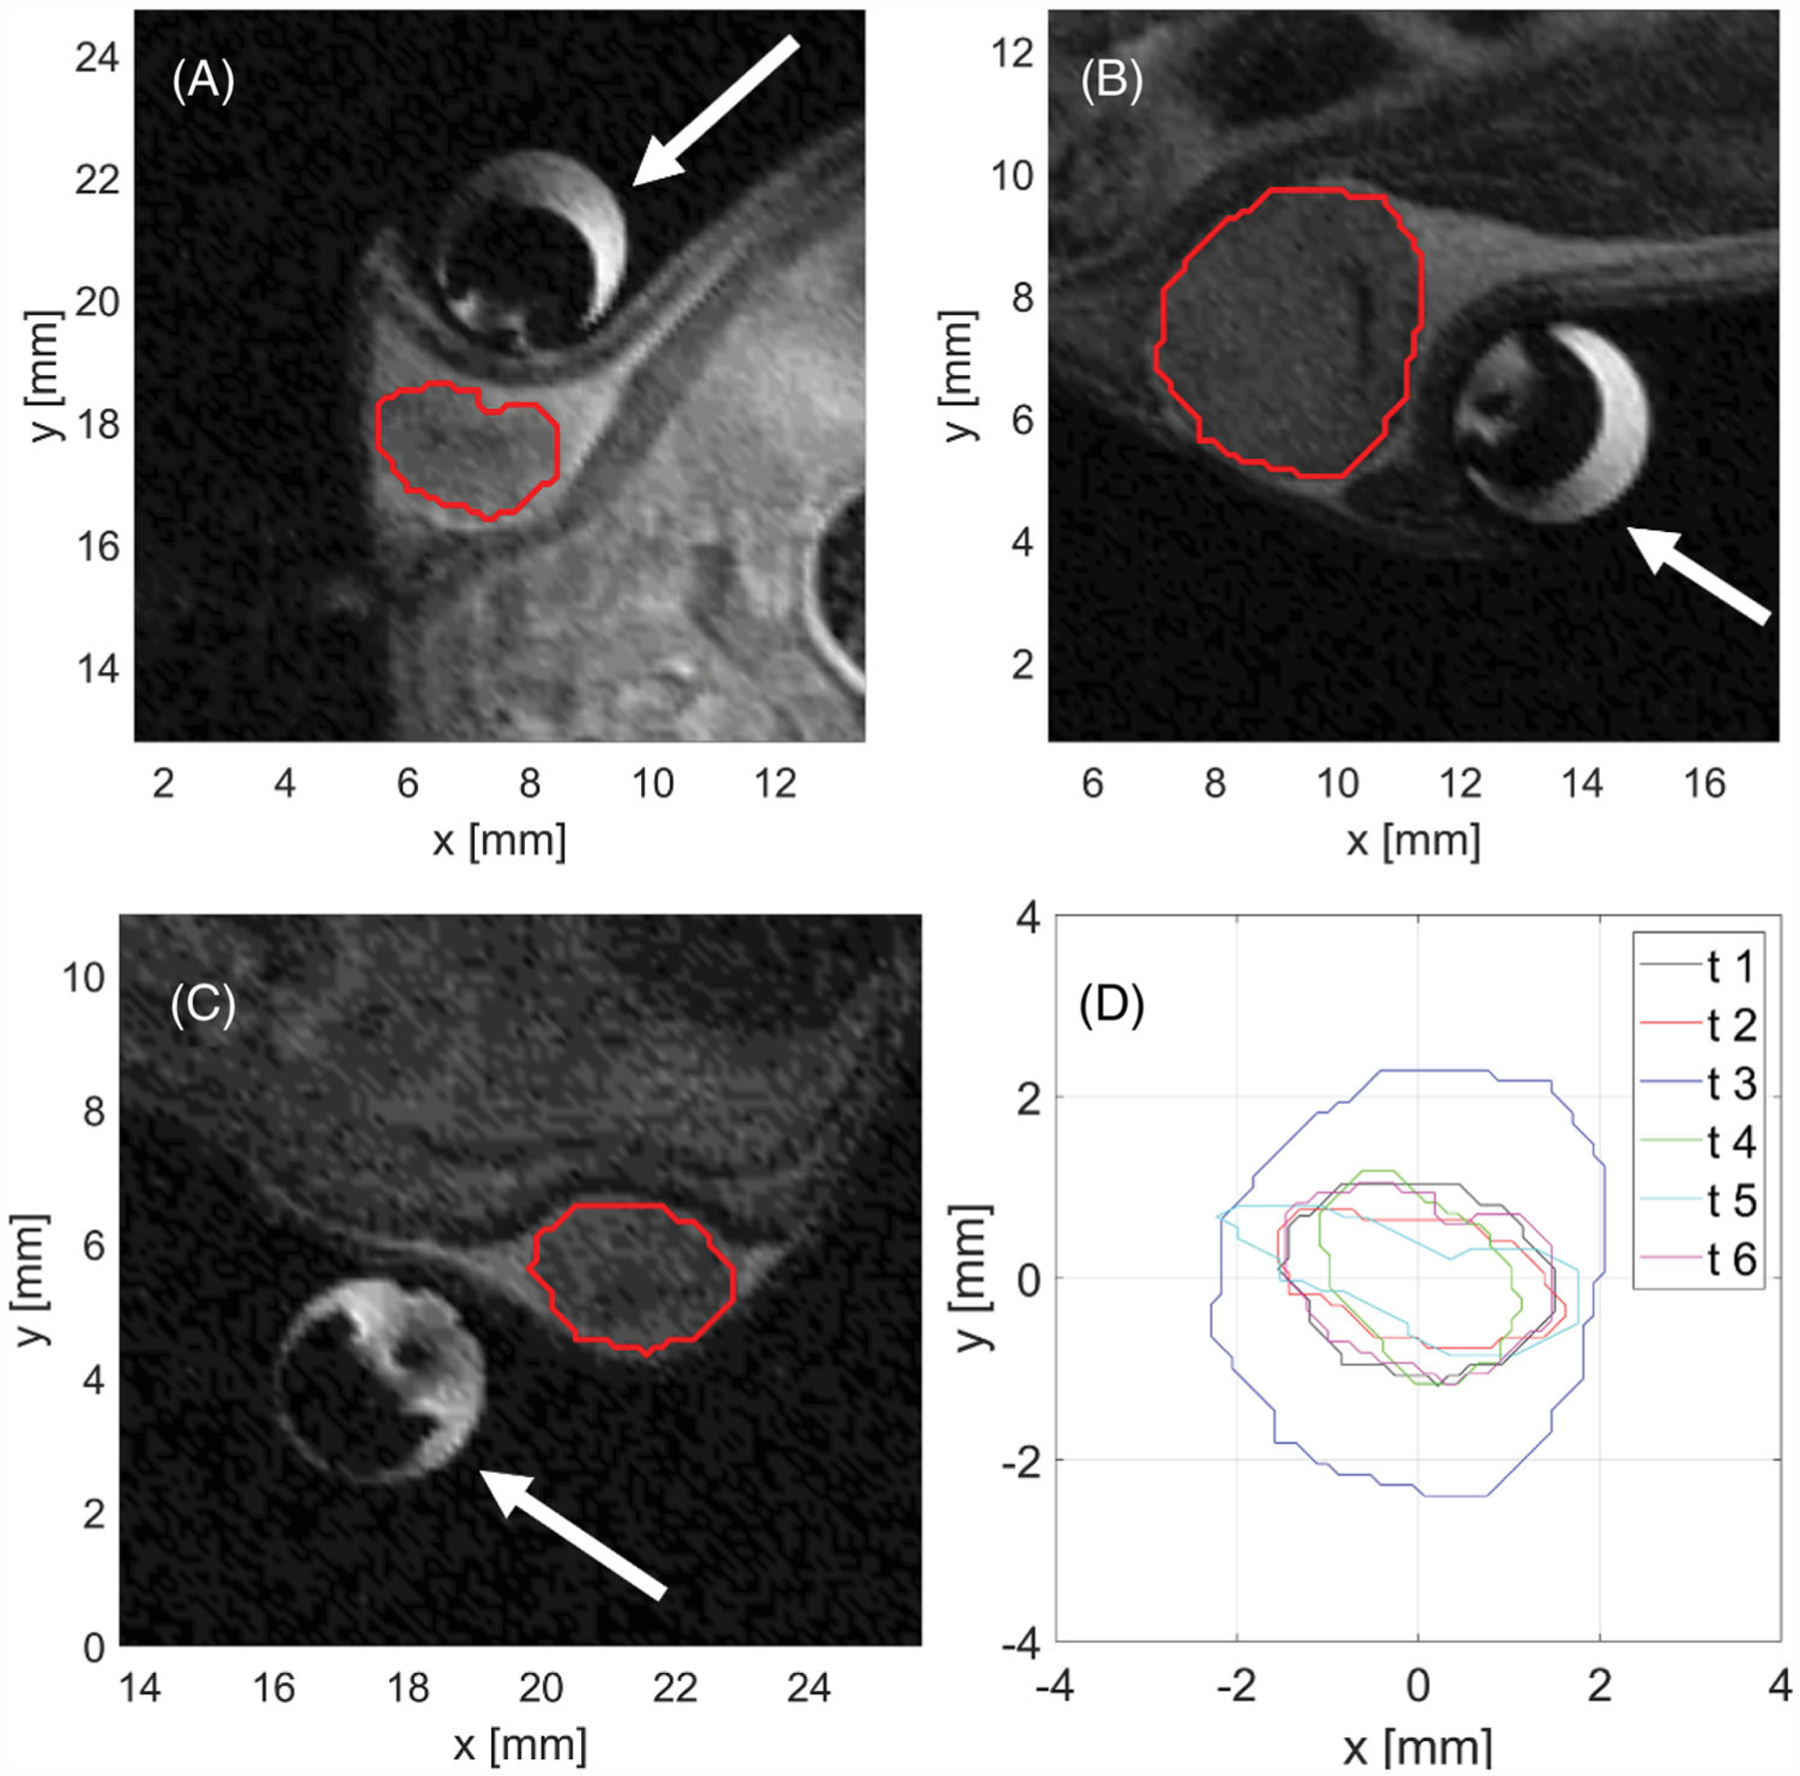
Example (A–C) anatomic MR images of subcutaneously implanted HAC15 tumors (red contour) and the microwave ablation applicator (white arrow). The boundaries of all six treated tumors (D) in a central axial plane through the tumor.
